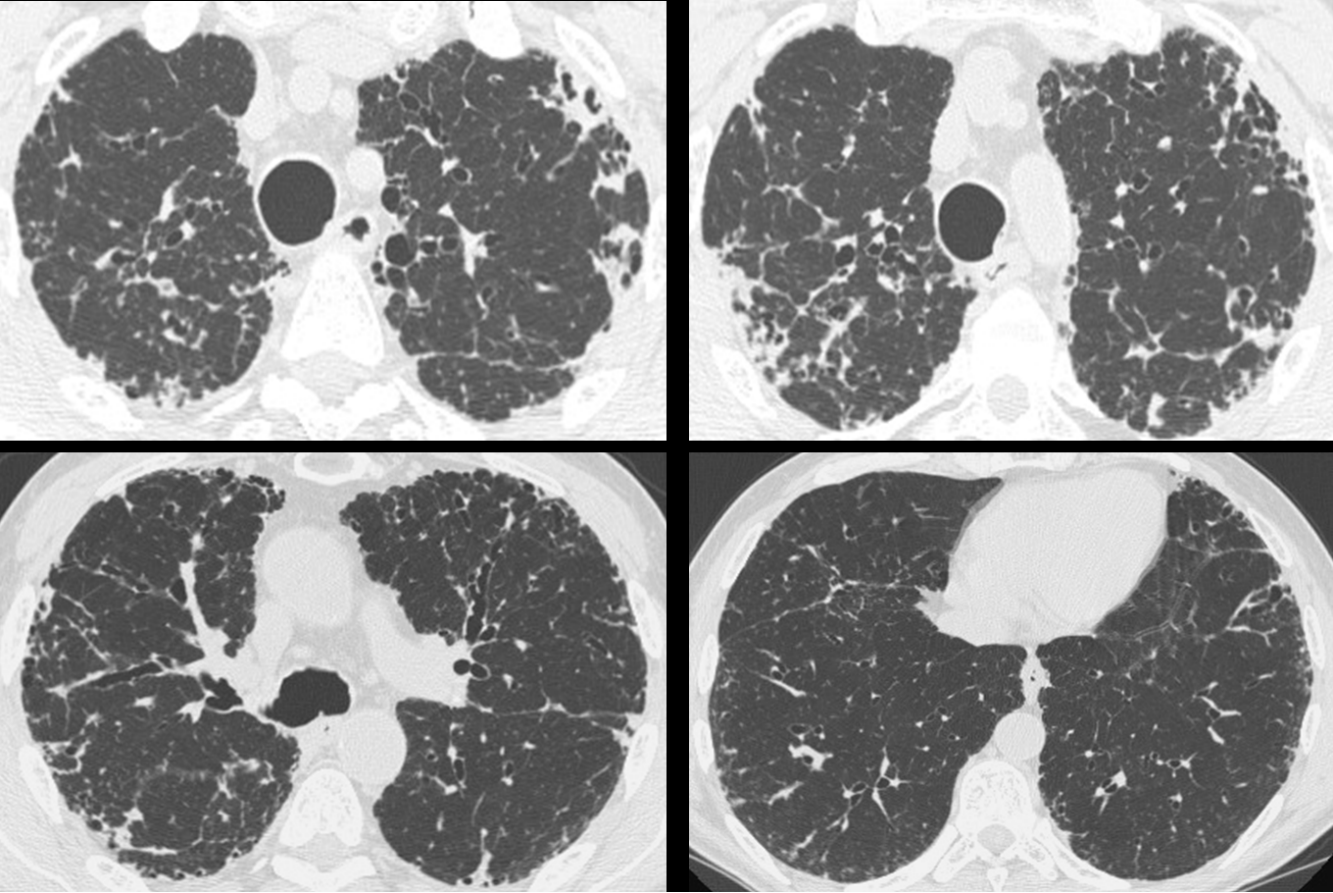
High resolution CT scan in patient no. 1 shows severe pleural and subpleural thickening with moderate fibrotic changes in the marginal parenchyma. Traction bronchiectasis and honeycombing are also seen.
